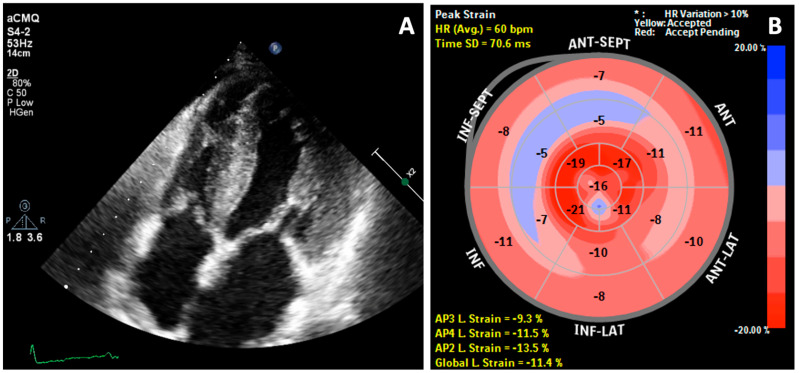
2D Transthoracic echocardiography: (A) Thickening of both atria, right ventricle walls, atrial septum, valves, and papillary muscles and (B) Reduced global longitudinal strain (GLS = −11.4%) with bull’s eye plot and pathognomonic pattern of relative apical sparing.
